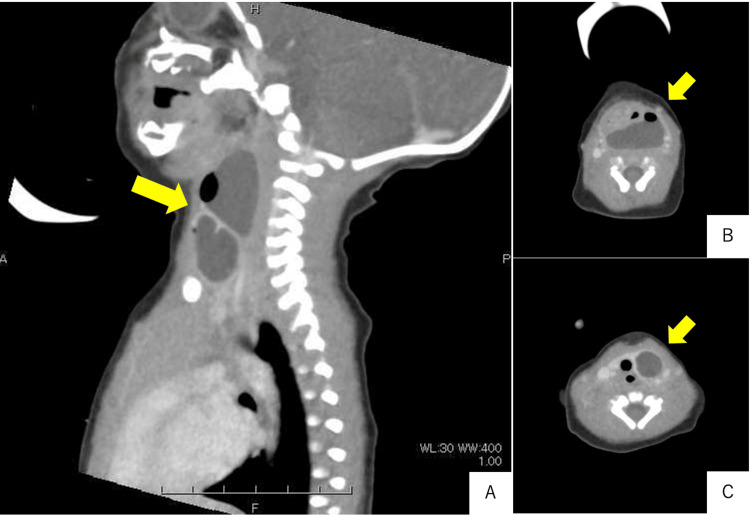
Contrast-enhanced CT indicating an air-containing cystic lesion extending from the pharynx to the neck (arrows) (A) Sagittal plane. (B) Axial plane, C4 level. (C) Axial plane, C6 level.
